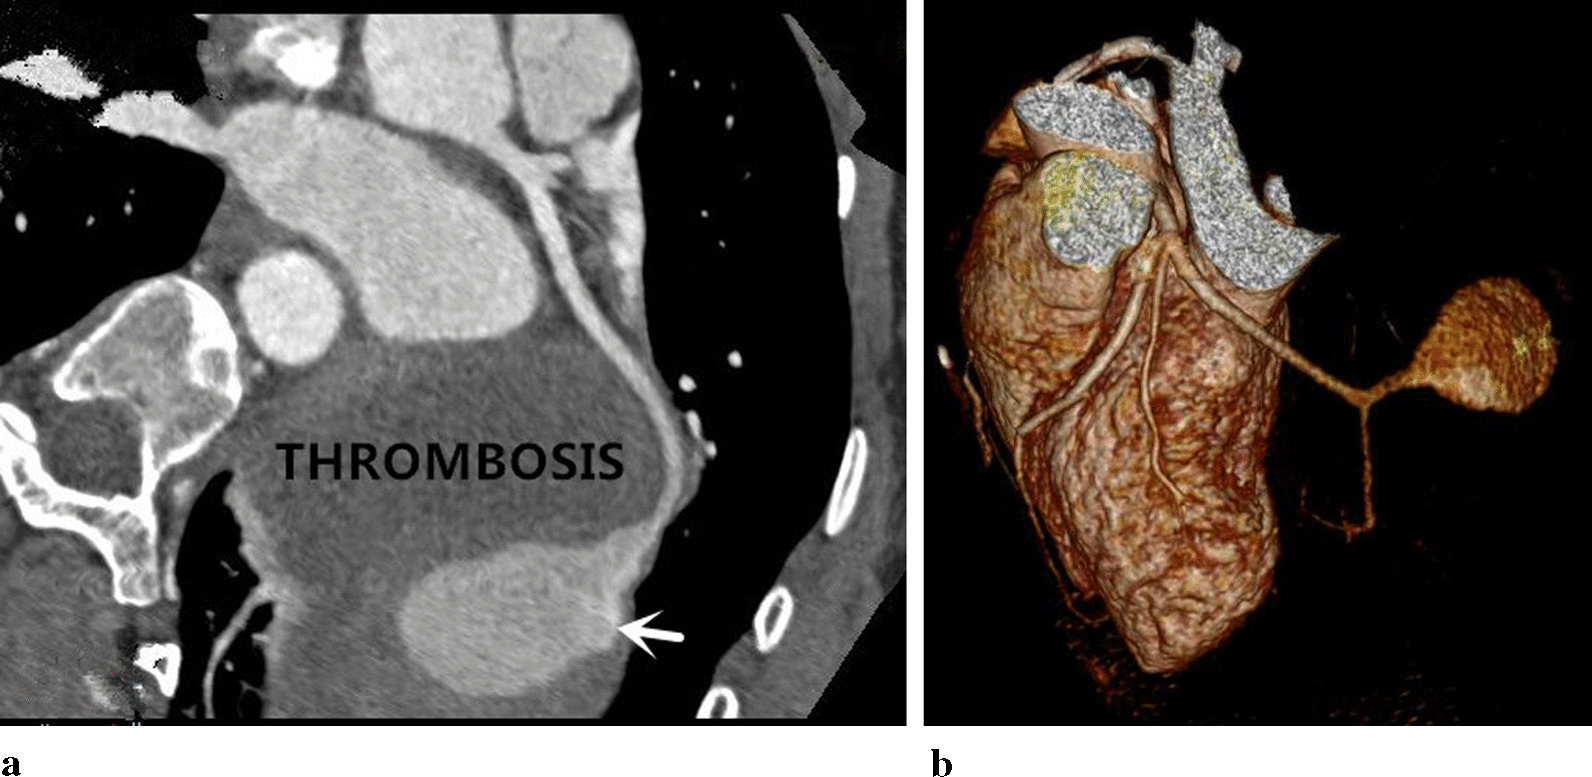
a Coronary computed tomography angiography (CCTA) revealed the giant LCX aneurysm with contrast agent filling observed in the lumen (white arrow) which contained huge intra-aneurysmal thrombosis. b Three-dimensional reconstruction showed the LCX distal pseudoaneurysm
